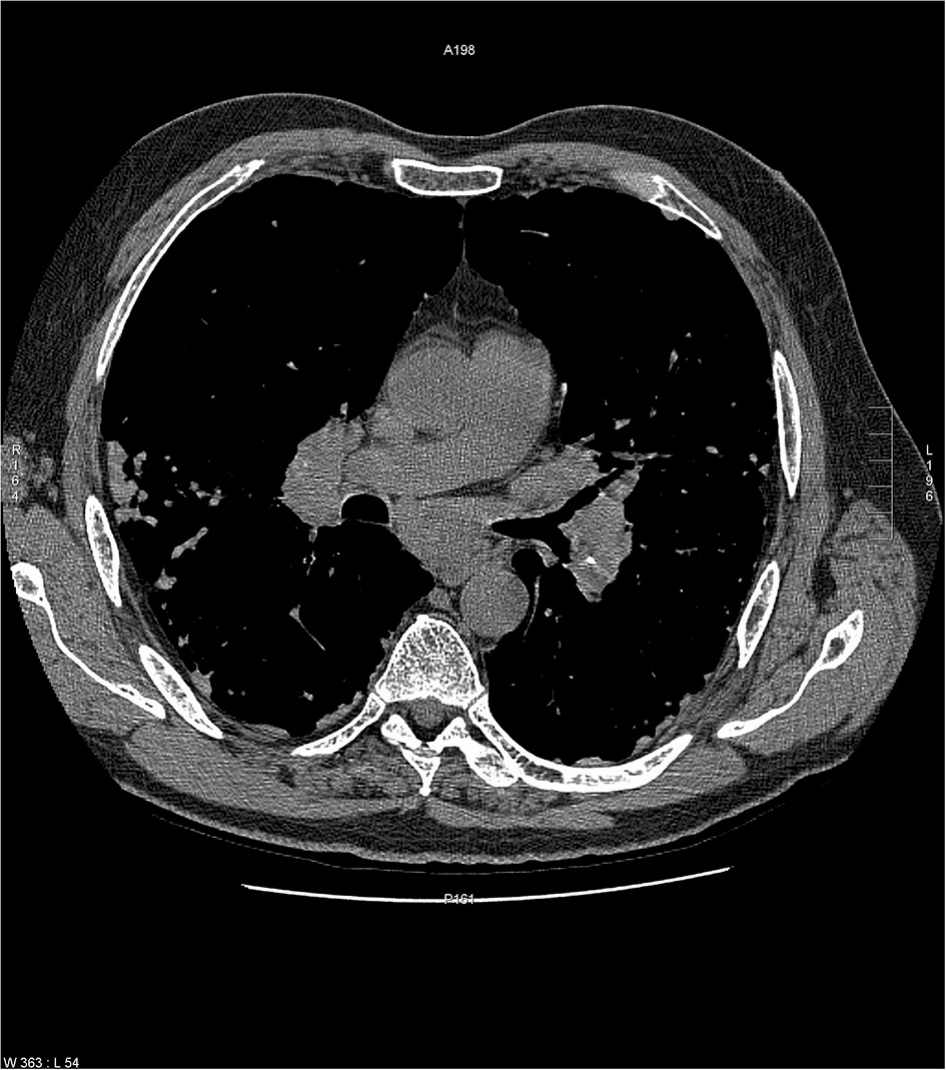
Chest radiograph of a patient with chronic beryllium disease showing imaging features typical of pulmonary sarcoidosis including bilateral hilar adenopathy with some calcium in lymph nodes. Reproduced with permission from Judson (52).
